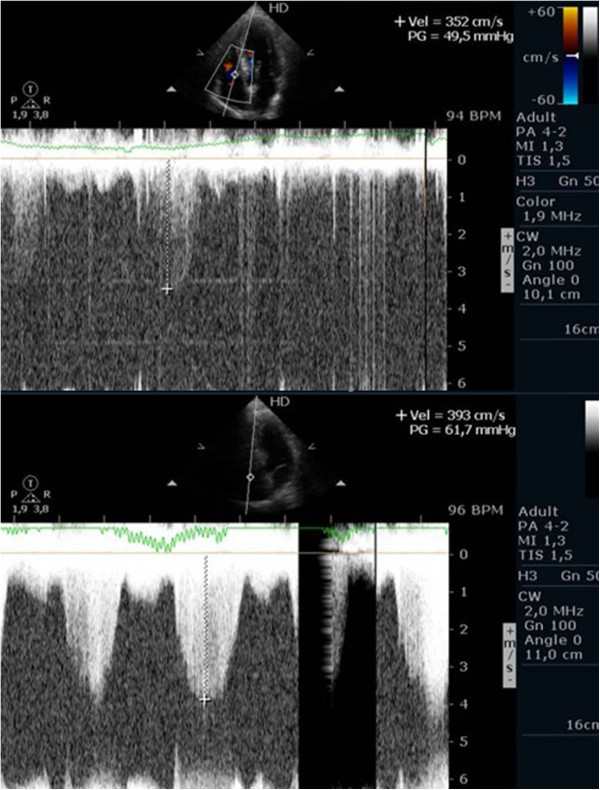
Continuous wave Doppler signal of the tricuspid regurgitant jet at peak exercise before and after injection of contrast.
